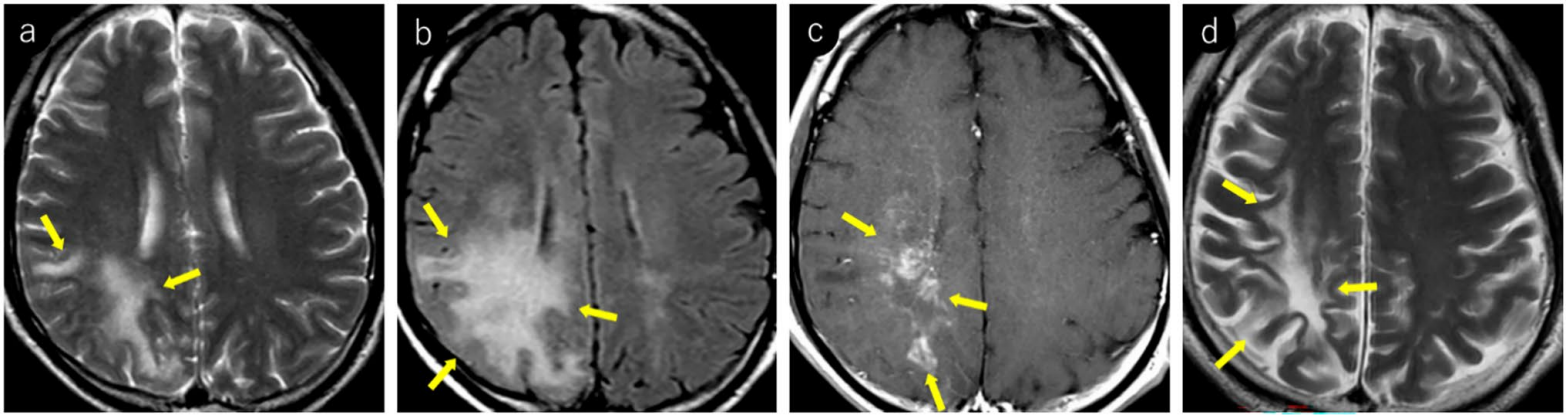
Previously untreated HIV-positive man in his 30 s presented with progressive visual impairment. a Initial MRI demonstrates T2 hyperintense lesions in the right occipitotemporal subcortical white matter (yellow arrows). CSF PCR confirms the diagnosis of PML. Two months after ART initiation: b FLAIR imaging showing the rapid expansion of existing PML lesions with mass effects and edematous changes (yellow arrows). c Post-contrast T1-weighted image showing patchy and punctate enhancement (yellow arrows). The combination of clinical and imaging findings led to the diagnosis of PML–IRIS. ART was continued, halting the progression of PML lesions. Long-term follow-up: d at the 10-year follow-up, the patient survived but showed marked brain atrophy in the affected regions (yellow arrows), with residual higher brain dysfunction and visual impairment
